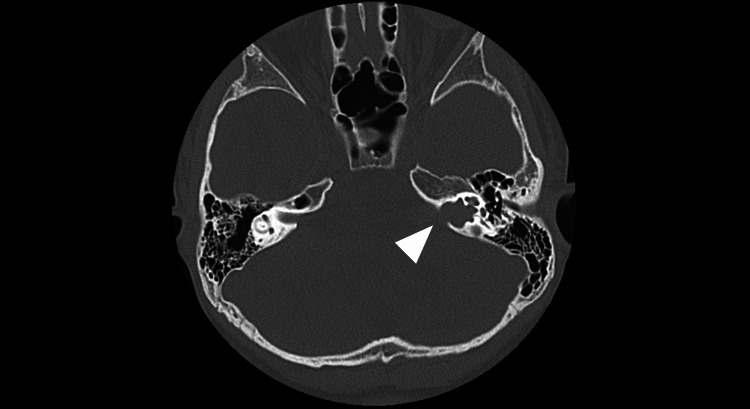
Preoperative CT scan of the skull base (bone window) Significant enlargement of the left internal auditory canal was observed (white arrowhead).
